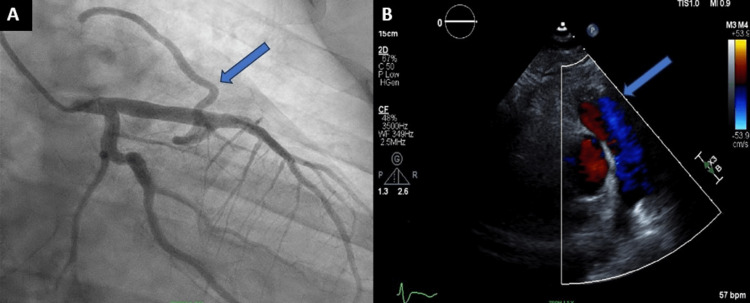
(A) Cardiac catheterization image showing mid-LAD to pulmonary artery fistula. (B) Doppler echocardiogram image showing the shunting. LAD - left anterior descending
